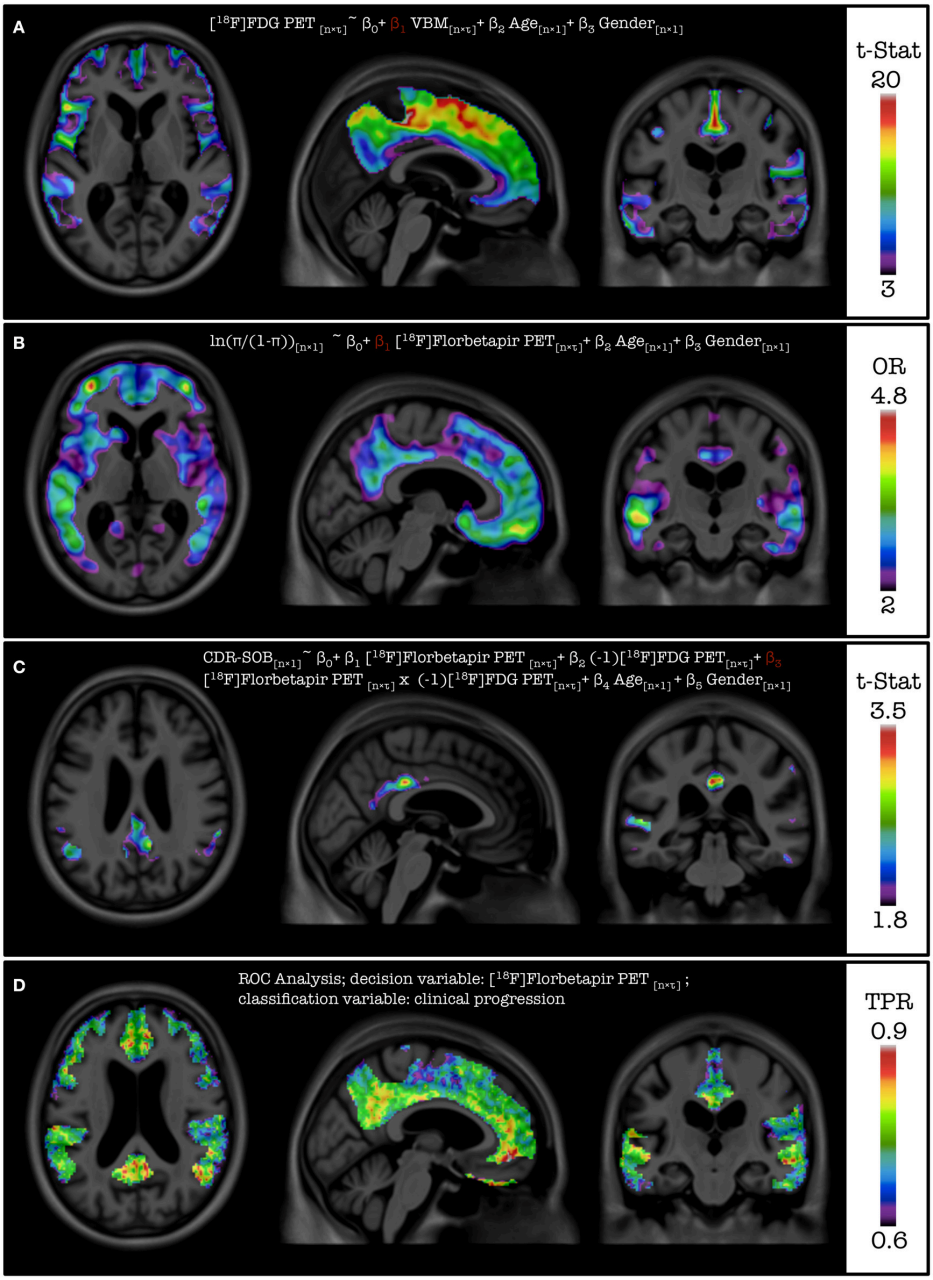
Results from the feature case assessments. (A) Multiple comparison corrected statistical significance of the parameter for VBM for the association with [^18^F]FDG PET. (B) Multiple comparison corrected scaled odds ratio values of developing dementia in 24 months for a unit increase of the standard deviation of [^18^F] Florbetapir PET SUVR. (C) Uncorrected statistical significance of the parameter for the interaction between [^18^F]Florbetapir PET and [^18^F] FDG PET for the association with CDR-SOB. (D) True positive rate values from the ROC analysis based on [^18^F] Florbetapir PET SUVR in classifying individual developing dementia in 24 months.
